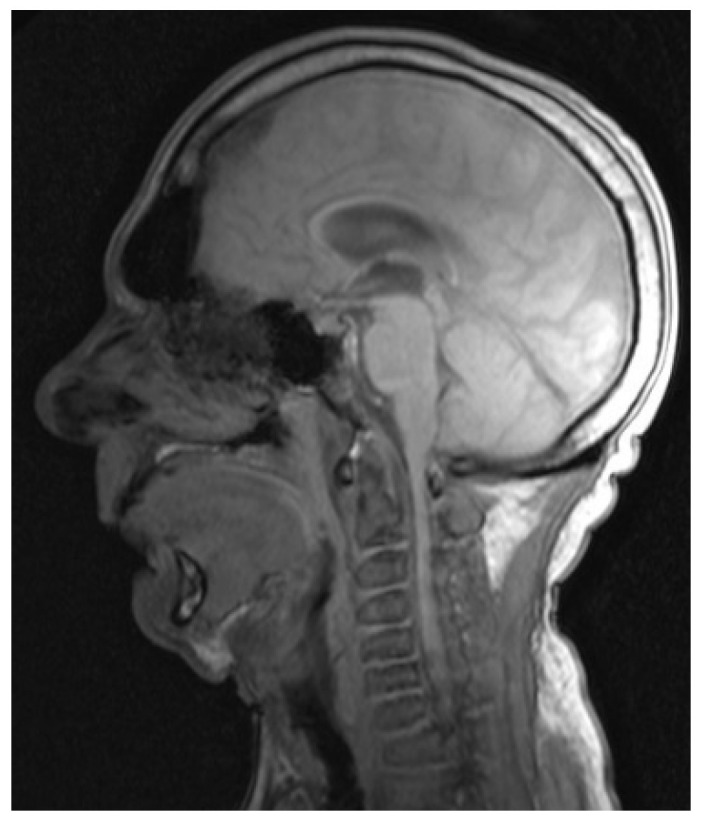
MRI scan of MPS I showing large tongue and high, anterior larynx, and absence of cervical lordosis.
